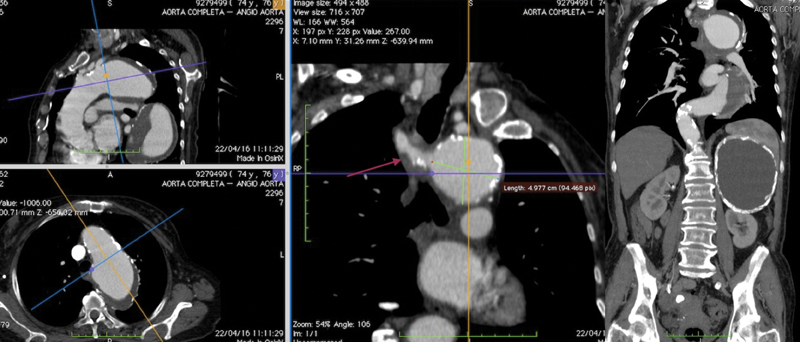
Computed tomographic angiogram showing the thoracic aortic aneurysm. Note the presence of the Kommerell diverticulum with the right aberrant subclavian artery and the huge cystic lesion of the left kidney.
